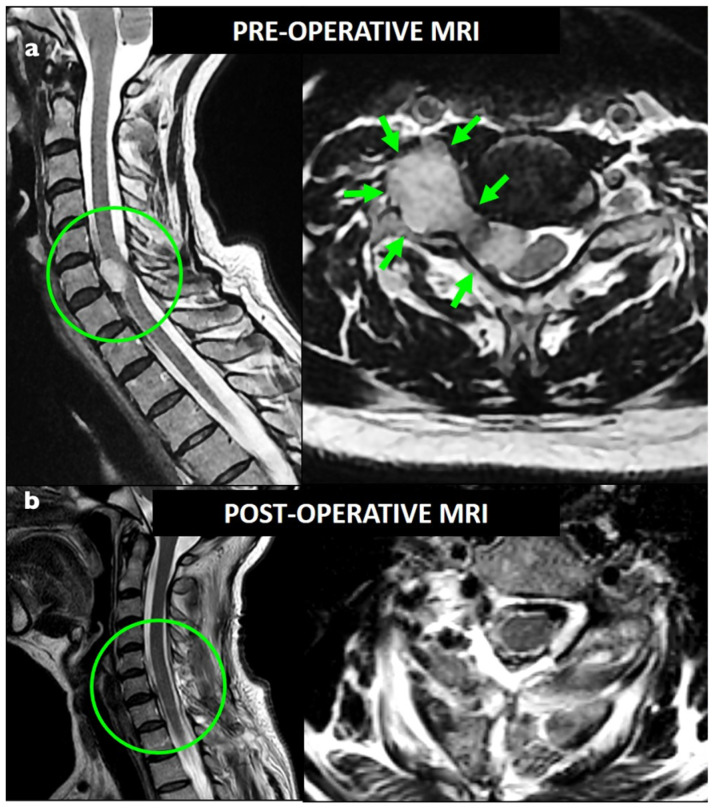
Preoperative (a) and postoperative (b) T2-weighted MRI showing a foraminal C7 dumbbell schwannoma (Sridhar class IVa); GTR was obtained with a single-stage posterior approach.
